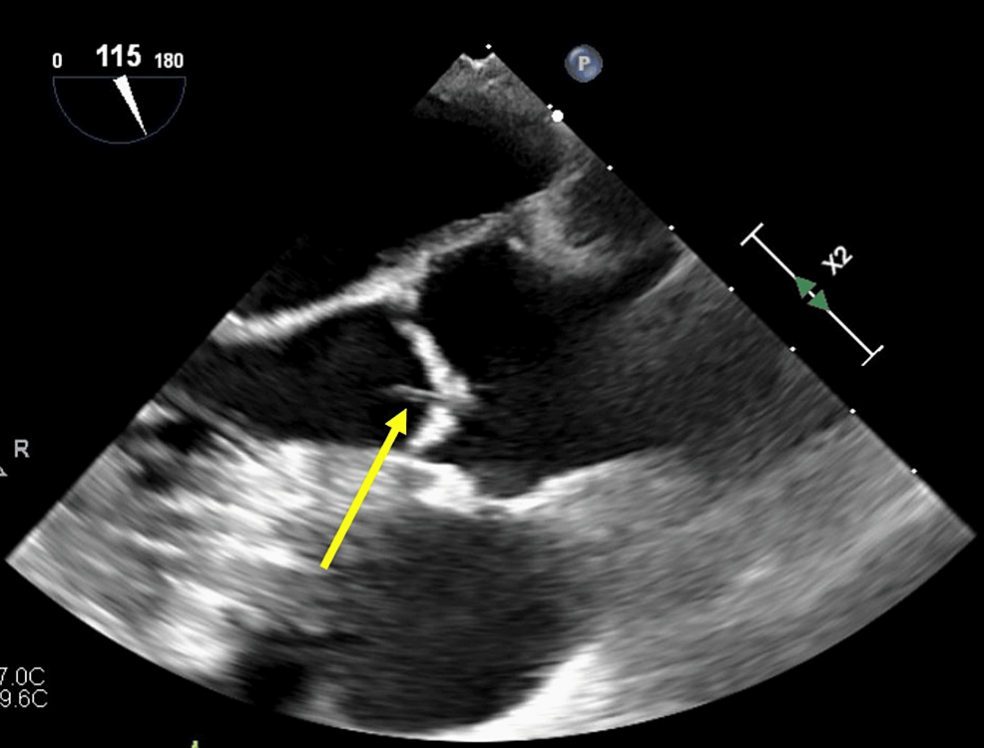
Transesophageal echocardiogram, midesophageal long-axis view Mobile filamentous structure attached to the aortic valve, consistent with Lambl’s excrescence (arrow).
